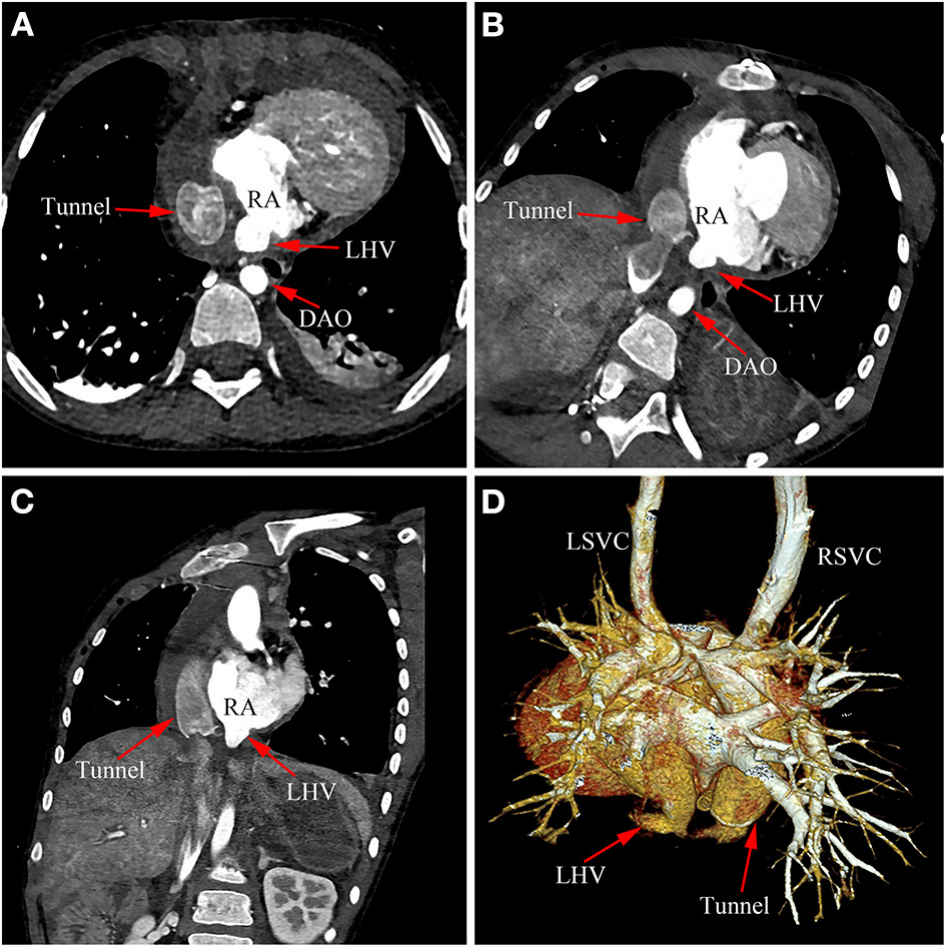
Cardiac CT image of anomalous left hepatic venous connection. (A–D) Postoperative CT image confirmed LHV came into the right atrium. LHV, left hepatic vein; DAO, descending aorta; RA, right atrium; RSVC, right superior vena cava; LSVC, left superior vena cava.
